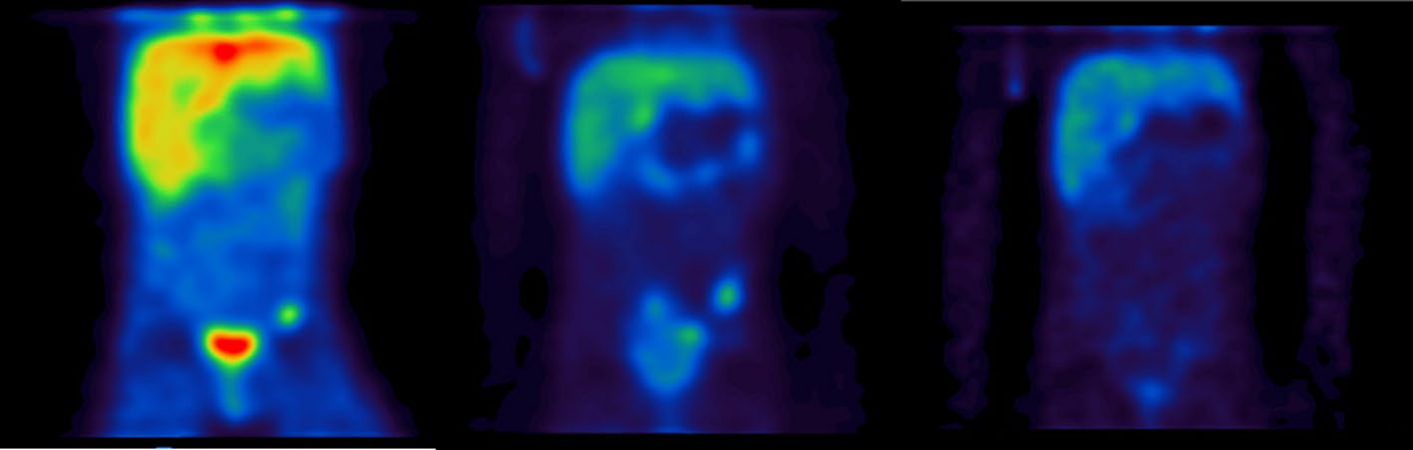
Coronal view of SPECT images at ~ 2 days, 5 days and 10 days. Quantification was performed on the bone metastases visualised in the left pelvic bone
